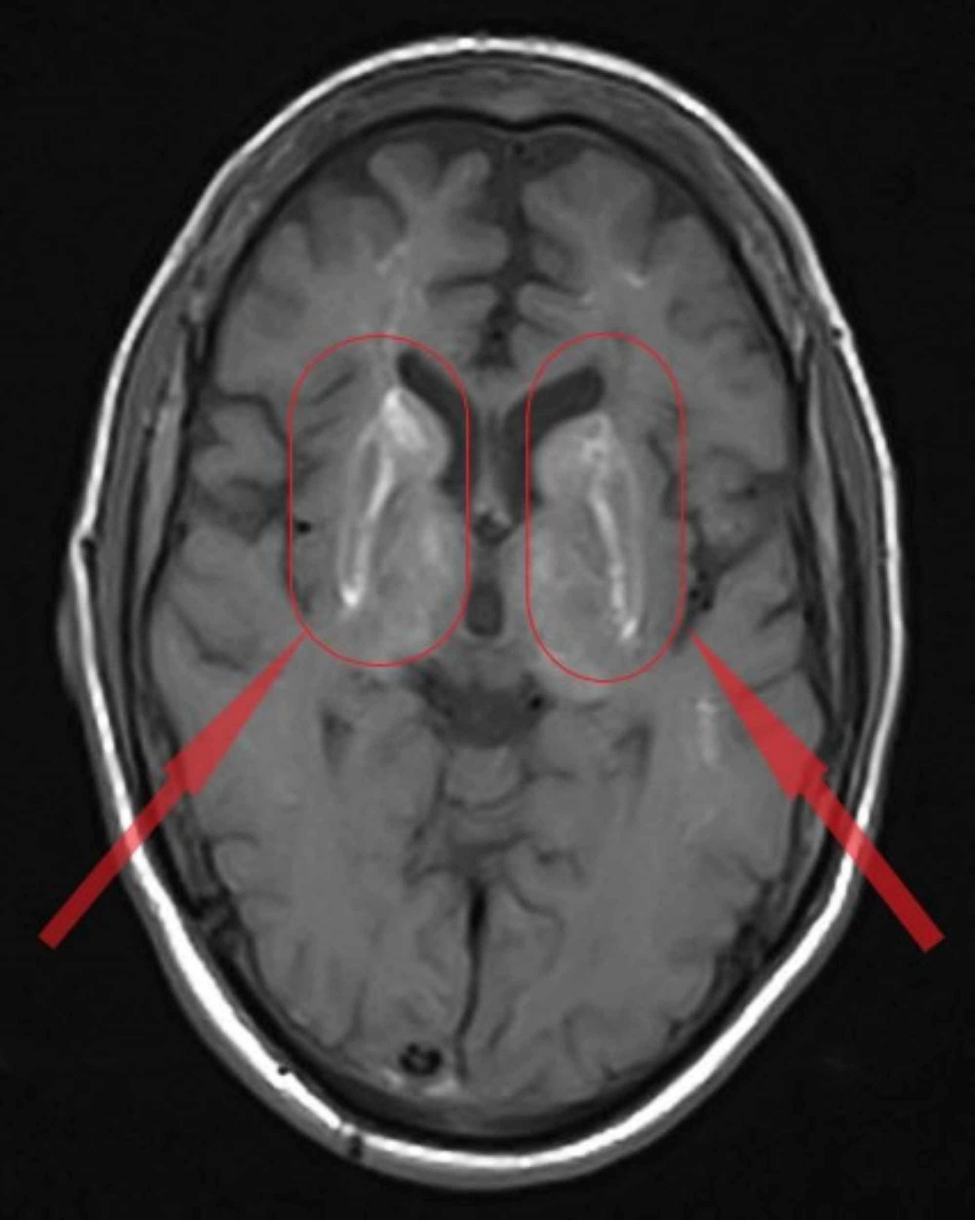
Basal ganglia calcification in the magnetic resonance imaging
